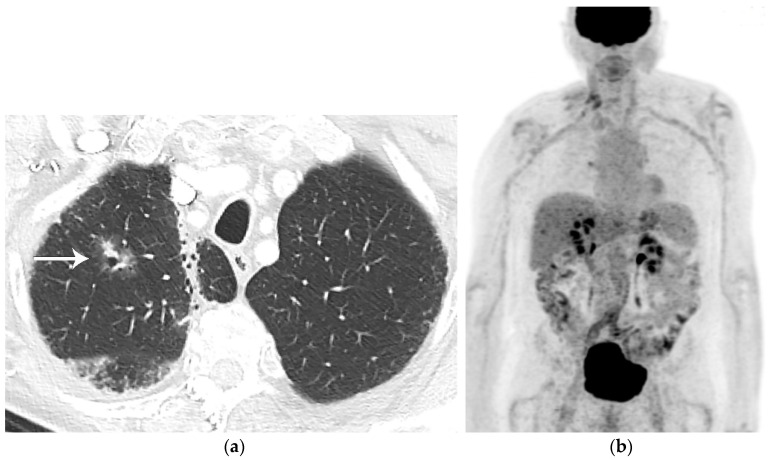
Lung adenocarcinoma. (a) CT shows a right upper lobe 2 cm part-solid nodule with focal “bubbly” internal lucencies and a solid component along the anterolateral aspect. Radiation fibrosis is noted in the right apex medially. (b) Whole-body PET shows the nodule is not FDG-avid. Biopsy revealed well-differentiated adenocarcinoma. Part-solid lung adenocarcinomas may not be FDG avid due to slow cell proliferation or poor cellularity.
